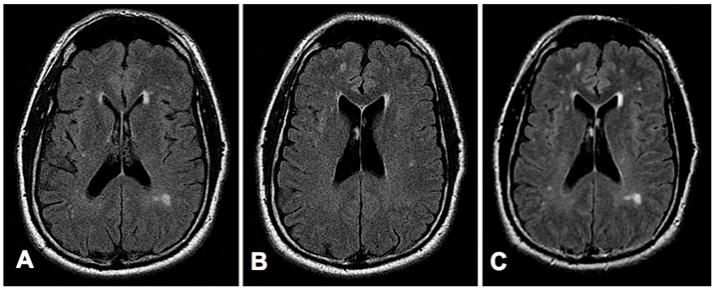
MRI Images obtained before (Panels A and B), and six months after (Panel C) the stem cell treatment of patient 1. Panels A and B: Consecutive axial FLuid-Attenuated Inversion Recovery (FLAIR) images through the lateral ventricles show multiple small foci of bright signal in the periventricular and subcortical white matter, consistent with plaques of multiple sclerosis. Panel C: Axial FLAIR image shows no significant change in the multiple periventricular and subcortical white-matter plaques. (For the comparison, note that this slice is positioned between those in A and B, and at slightly different scanning-angle, so it includes lesions of both those slices, as well as others slightly out-of their plane.).
